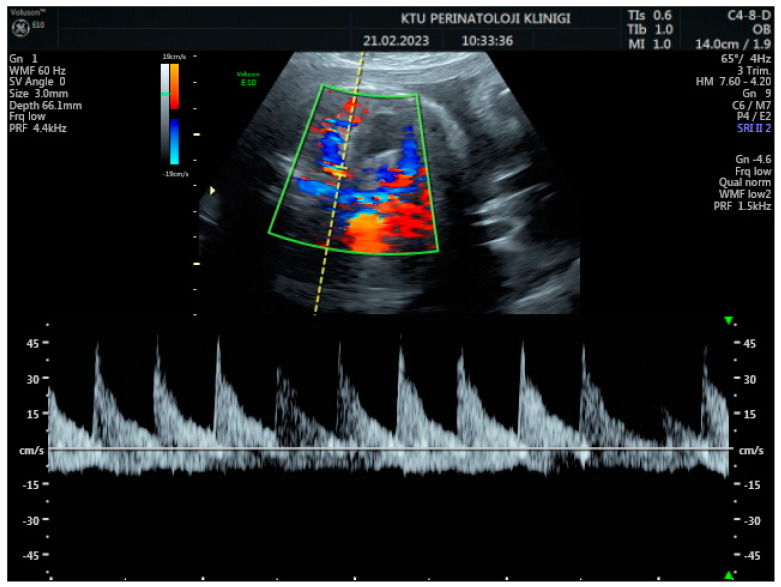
Two-dimensional ultrasonographic imaging of the 30-week-old fetus shows pulsed Doppler waveforms of the splenic artery.
